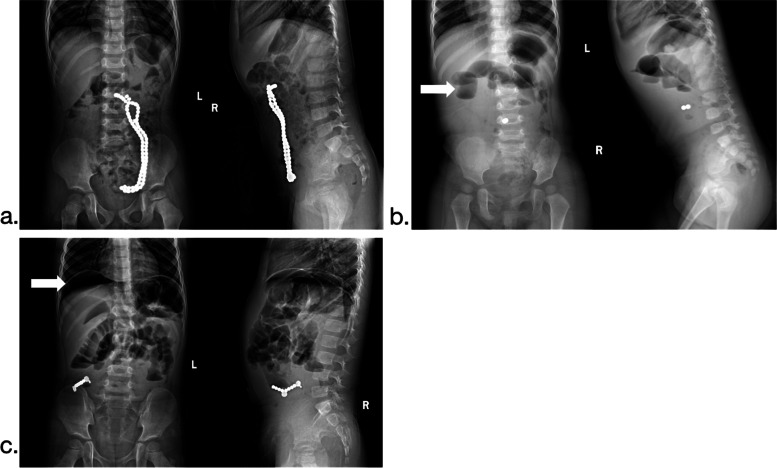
a Numerous magnetic foreign bodies, as determined by a multi-angle chest and abdomen X-ray. b Intestinal obstruction caused by magnetic foreign bodies with dilated proximal small bowel loops (white arrow). c Gastrointestinal perforation caused by magnetic foreign bodies, with sub-diaphragm free gas (white arrow)
